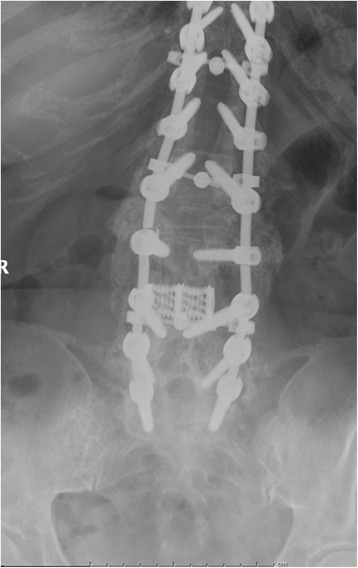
AP X-rays demonstrating fracture of rod at osteotomy site
